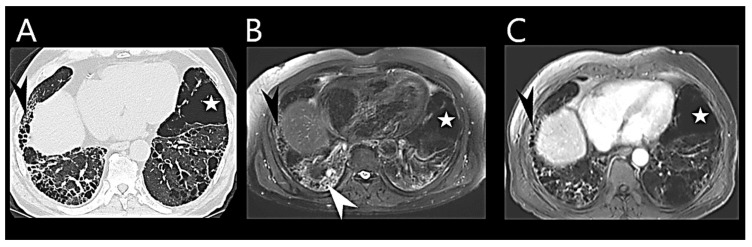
Cardiovascular magnetic resonance imaging (MRI) in idiopathic pulmonary fibrosis. Comparison of radiological findings in idiopathic pulmonary fibrosis with thin-slices multi-detector computed tomography (MDCT) and MRI. Thin-slices MDCT (A), MRI STIR (Shot Tau Inversion Recovery) sequence (B), and T1-weighted contrast-enhanced administration (at 10 min.) (C). The magnified images of MDCT and MRI show reticulation and honeycombing (black arrow) as well as areas of emphysema (white star). The high signal of ground-glass opacity in MRI (white arrow, (B)) may be due to water content and indicates active inflammation (white arrow, (B)).
